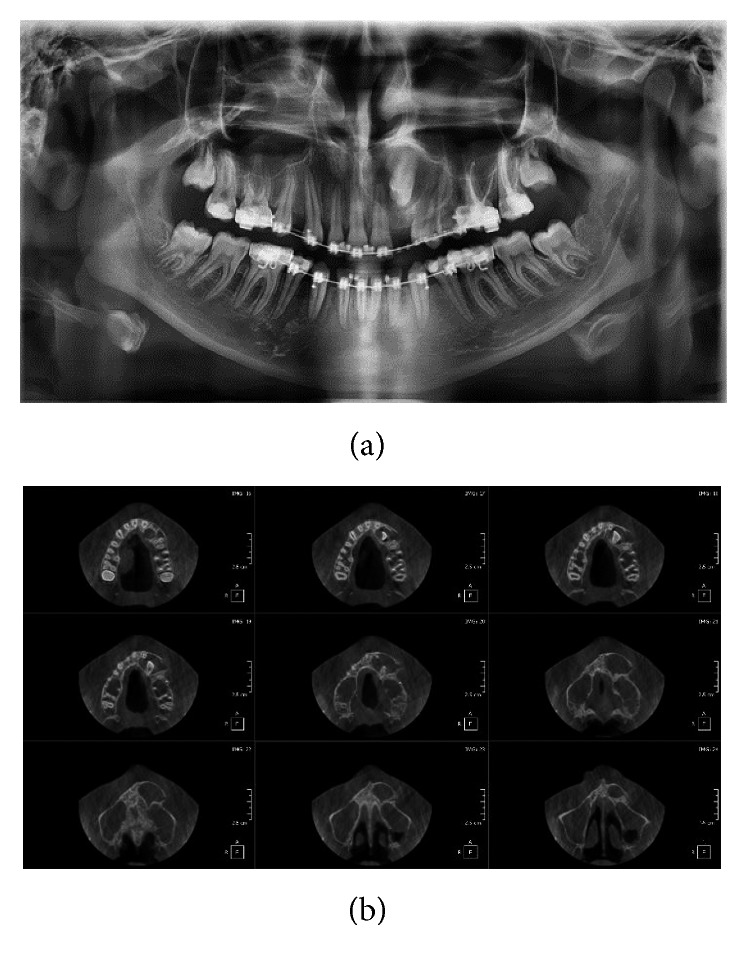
(a) Orthopantomogram and (b) axial view of cone beam computerized tomography, showing a well pericoronal circumscribed radiolucent image associated with impacted tooth #22.
